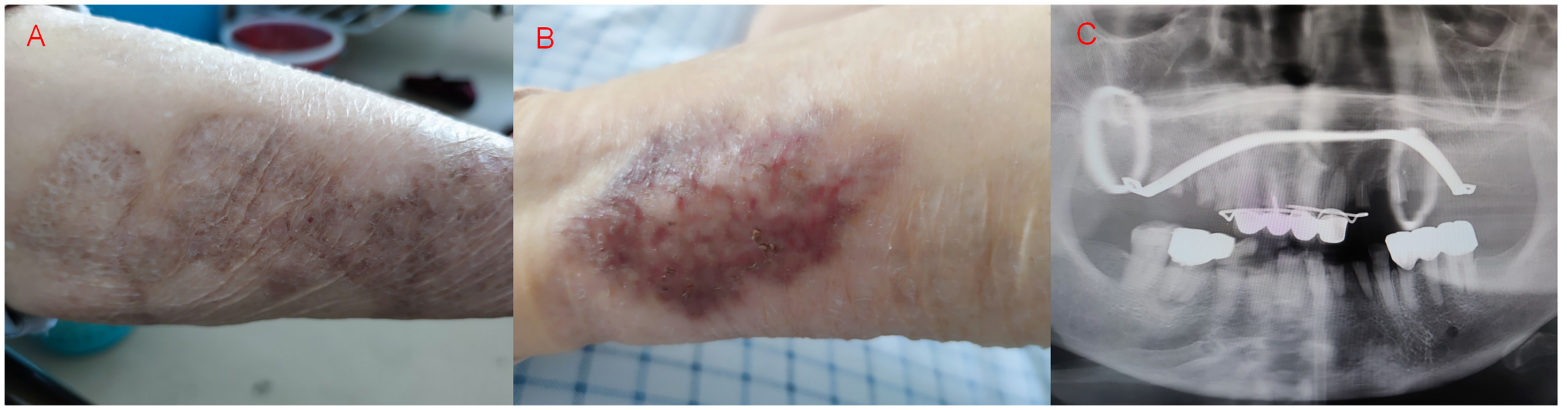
Skin damage of the patient's left lower extremity and dental plain radiographs, (A, B) Neovascularization, crusting, flaking, and hyperpigmentation were seen on the patient's left lower extremity skin, (C) Patient with multiple chipped and destroyed teeth, artificial crown and bracket placement visible.
